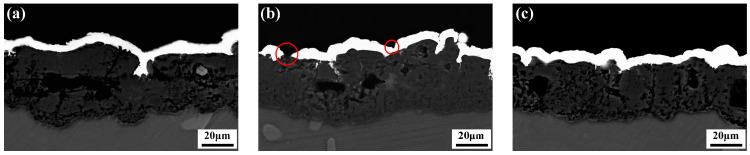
Cross-section observations of coatings after immersion in alkaline bath for 30 min with different activation methods. (a) 2 g/L AgNO_3_; (b) 10 g/L AgNO_3_; (c) 10 g/L AgNO_3_+ Ultrasonic.
